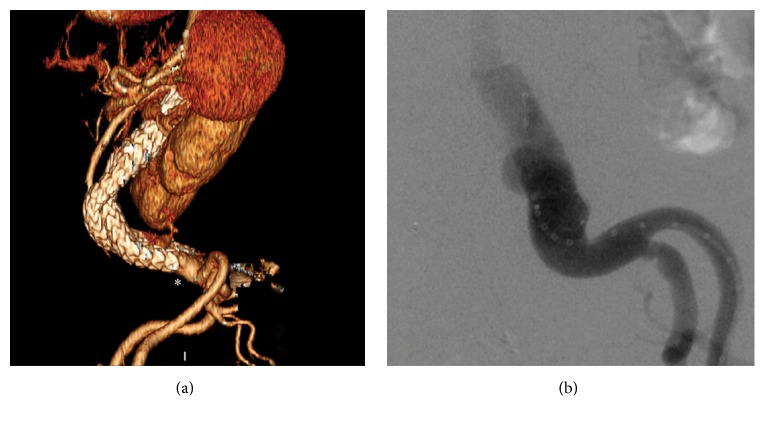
Follow-up at 12 months demonstrates a proximal migration of the left endograft limb (asterisk) on three-dimensional reconstruction of the CTA (a), leading to a type 1b endoleak as demonstrated in the aortogram (b).
